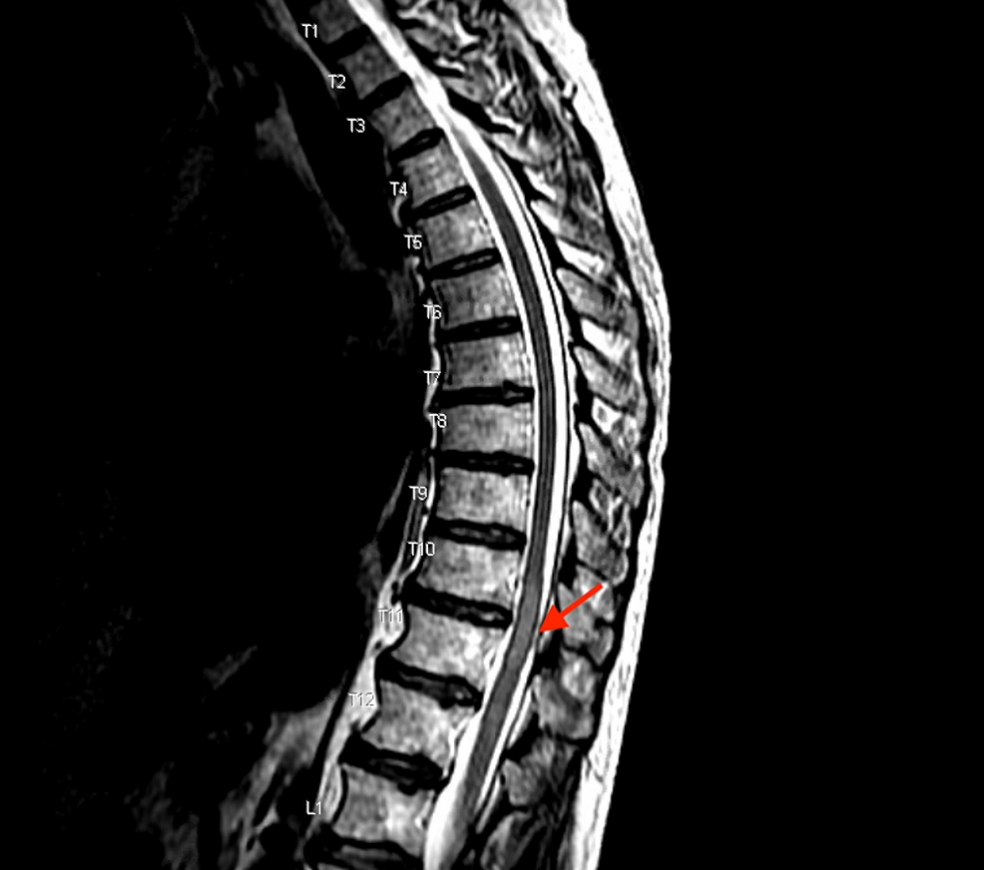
MRI of thoracic spine, T2 sagittal view. A slight hyperintense signal on T2 weighted sequence can be visualized.
